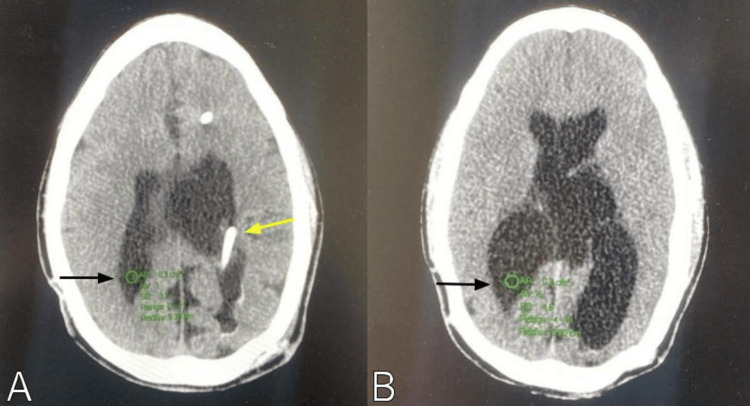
Axial head CT scan images showing the 0.3 cm radius region of interest circle at the patient's lateral ventricle atrium (black arrow). (A) Measurement is done at the ventricle contralateral to the shunt catheter, which is marked by the yellow arrow. (B) The circle is placed in an area without choroid plexus.
